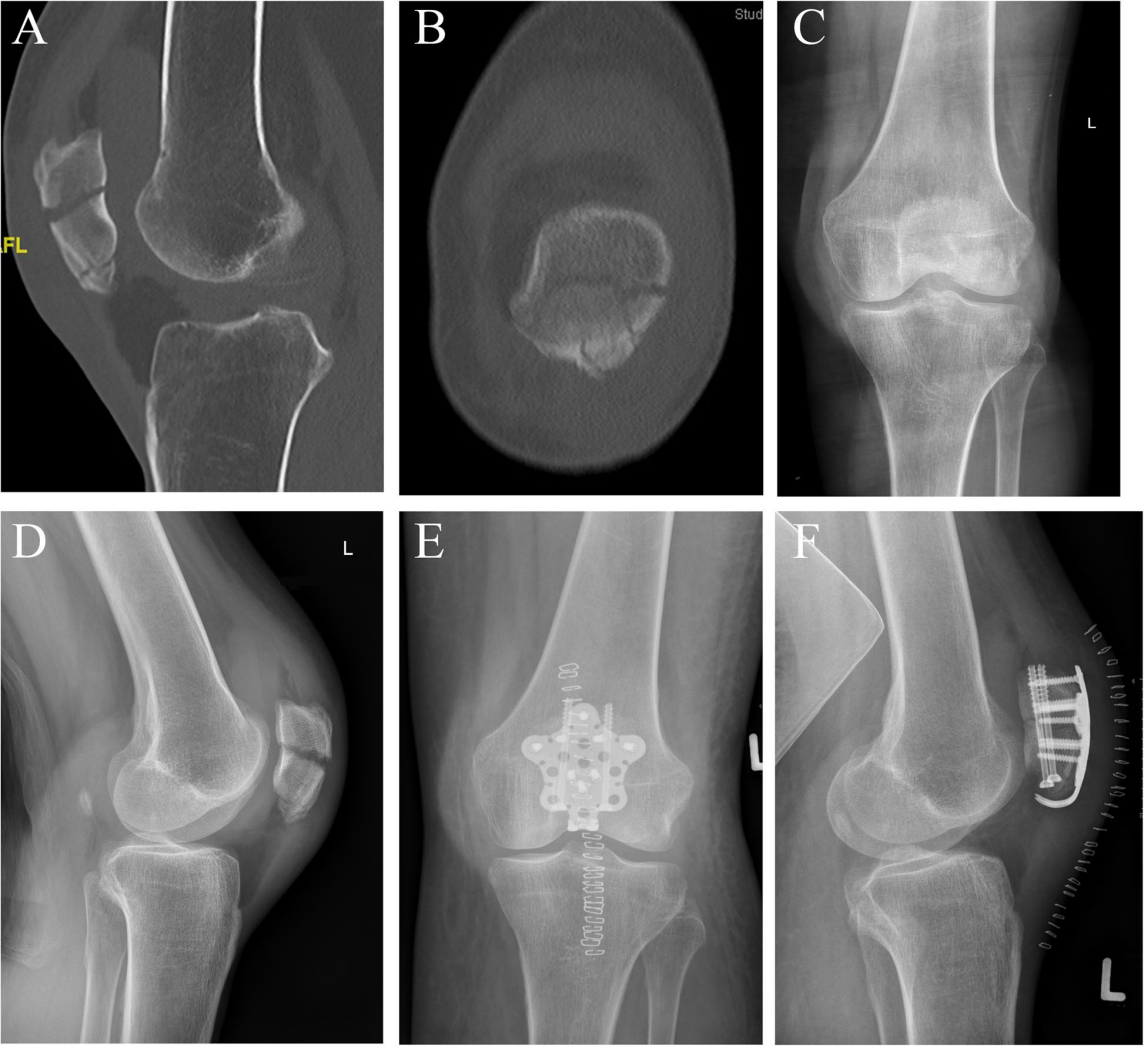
a-f: patella fracture with involvement of the inferior pole, overlooked in initial radiography but diagnosed in a preoperative CT (a—d). Postoperative X-ray shows an anatomic reduction with a locked hook plate and compression screws (e–f)
